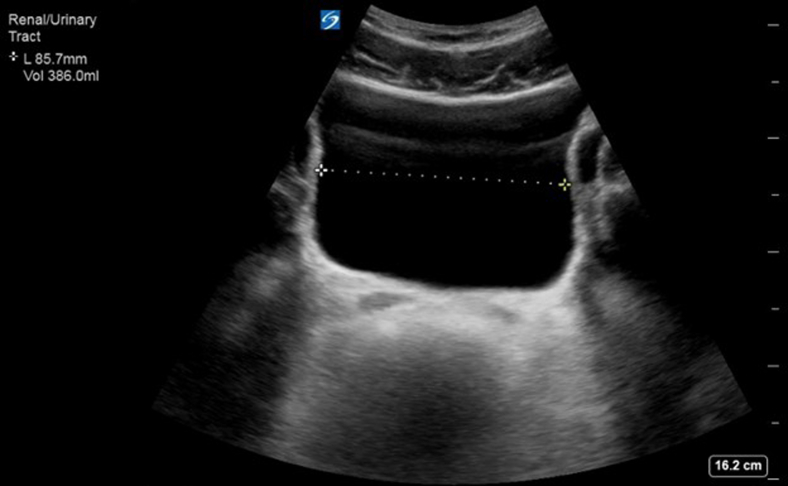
Ultrasound of postvoid bladder.
